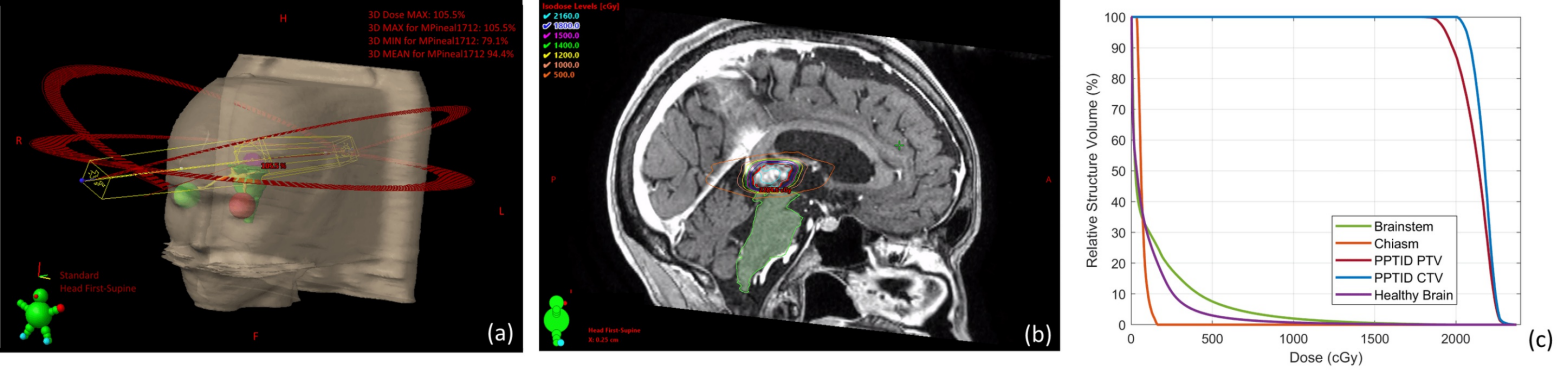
Patient treatment plan with three non-coplanar low couch angle arcs (a); dose distribution overlaid on the magnetic resonance imaging (MRI) with the clinical target volume (CTV) contour in red and the brainstem in green (b); dose-volume histogram (DVH) of relevant structures (c).
